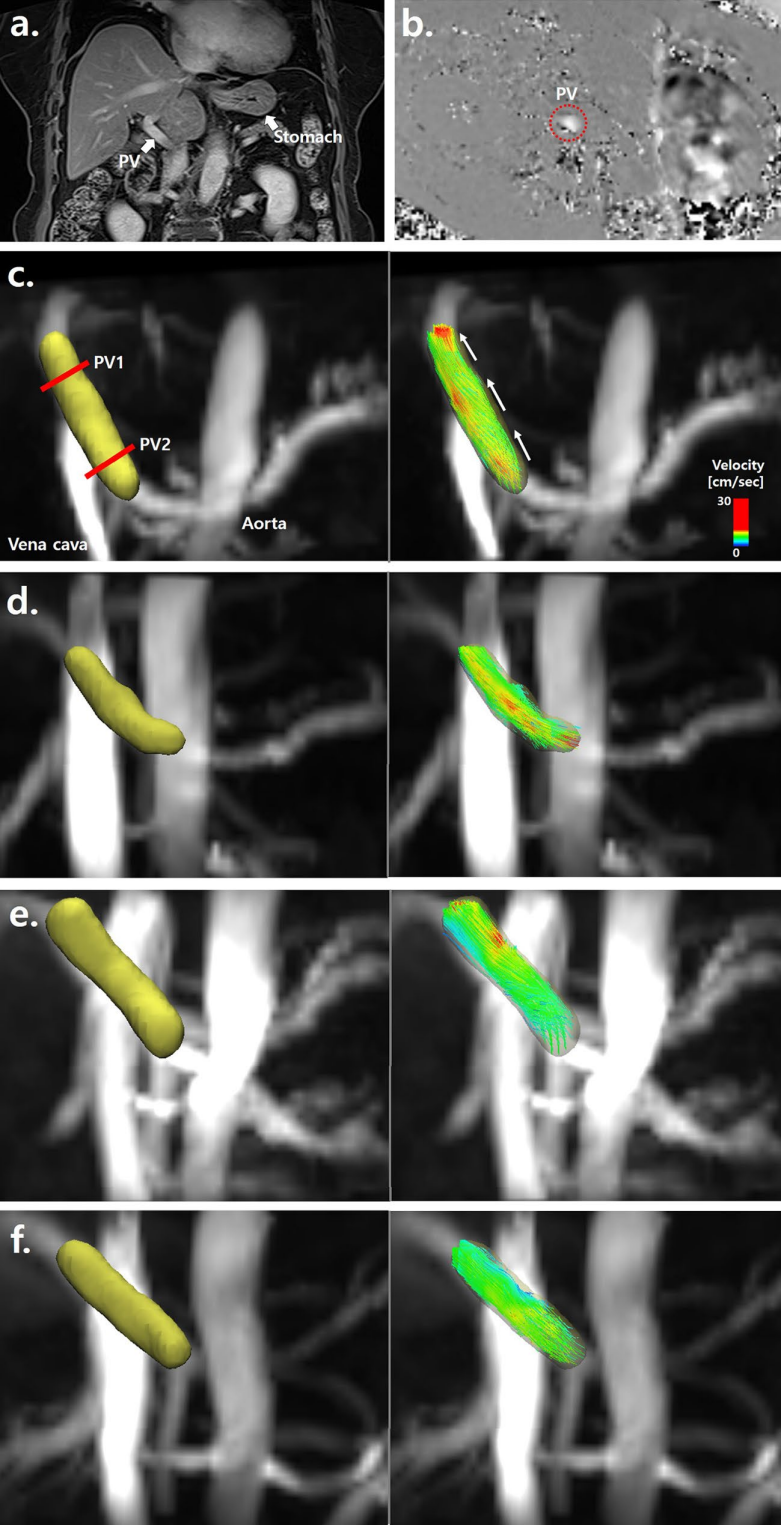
Anatomic coronal MR image (a) in the abdomen showing the main portal vein (PV) and the status of the stomach. Velocity-encoding (VENC) image (b) focusing on the PV (VENC = 30 cm/s). The red dotted circle indicates the cross-sectional area of the PV. Four-dimensional flow MRI data were post-processed; then, segmented anatomic images (left) and color-coded streamline images (right) were generated from a 38-year-old male (c), a 45-year-old female (d), a 53-year-old male (e), and a 68-year-old female (f). The red solid lines in (c) indicate planes for measuring the hemodynamic parameters, and the white arrows in (c) show the direction of blood flow.
